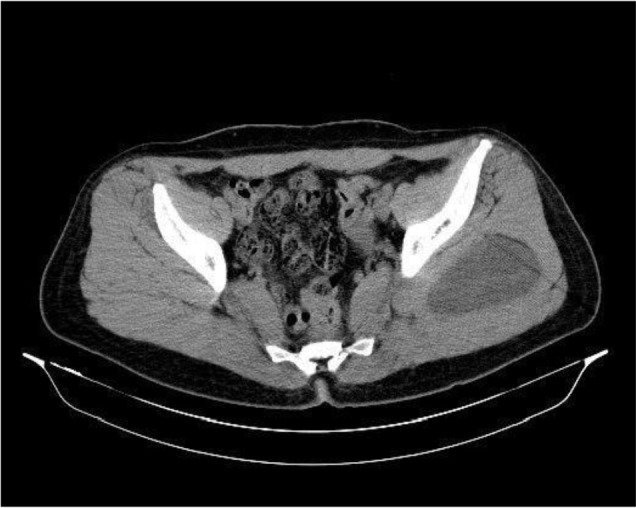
CT scan demonstrated cystic lesion in the left gluteus
